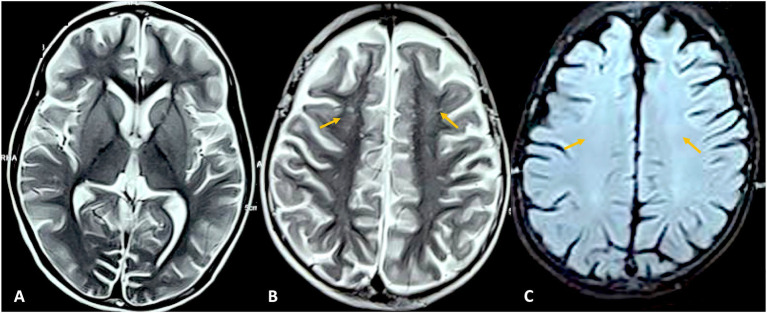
Neuroimaging in MMACHC. MRI brain axial sections T2-weighted sequences (A) showing diffuse brain atrophy, sparing of basal ganglia, and T2-weighted (B) and FLAIR (C) sequences showing hyperintensities in the fronto-parietal white matter bilaterally in a child with MMACHC.
